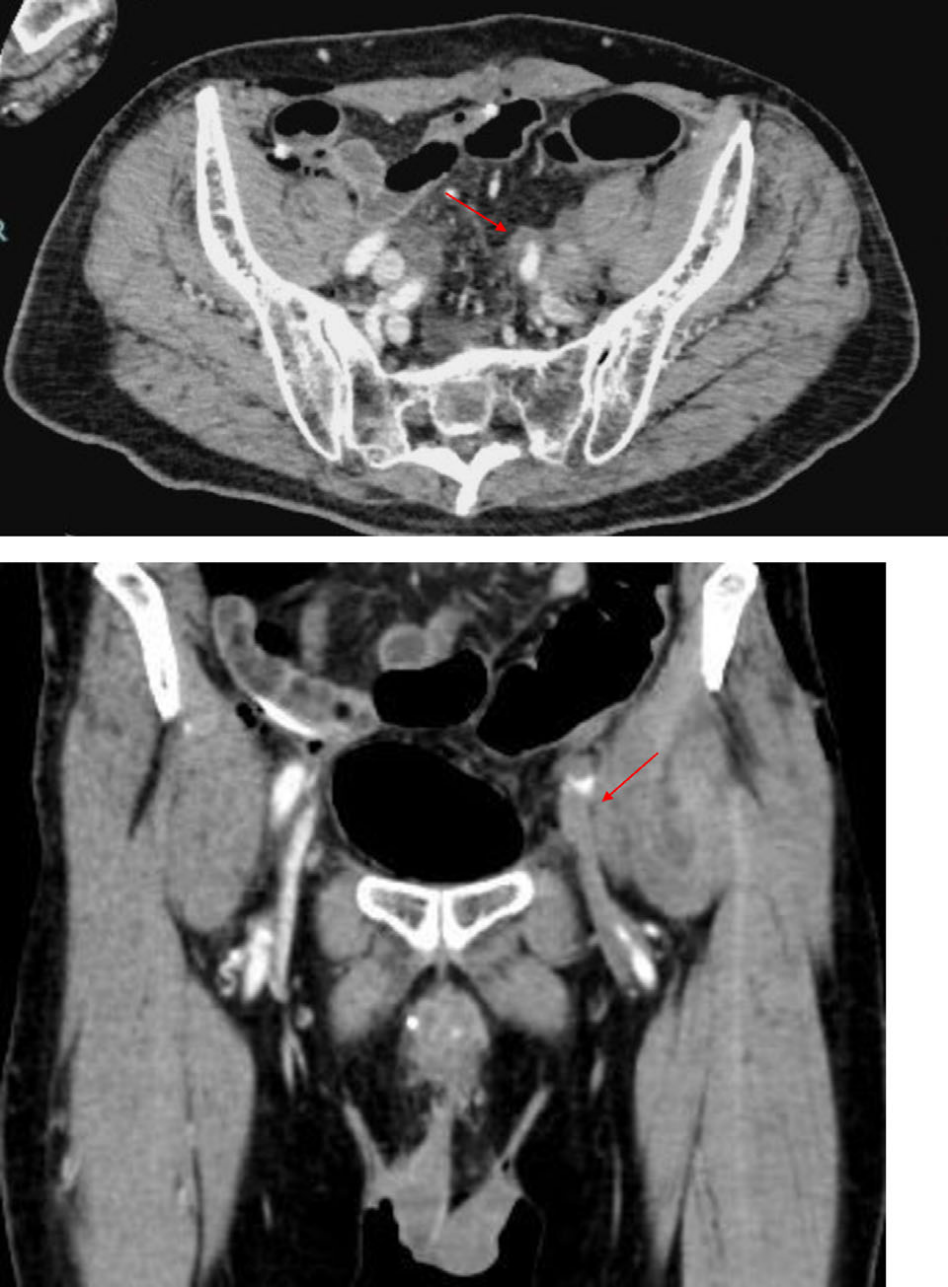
Arrow in CT image shows an external iliac arterial dissection and thrombotic occlusion.
